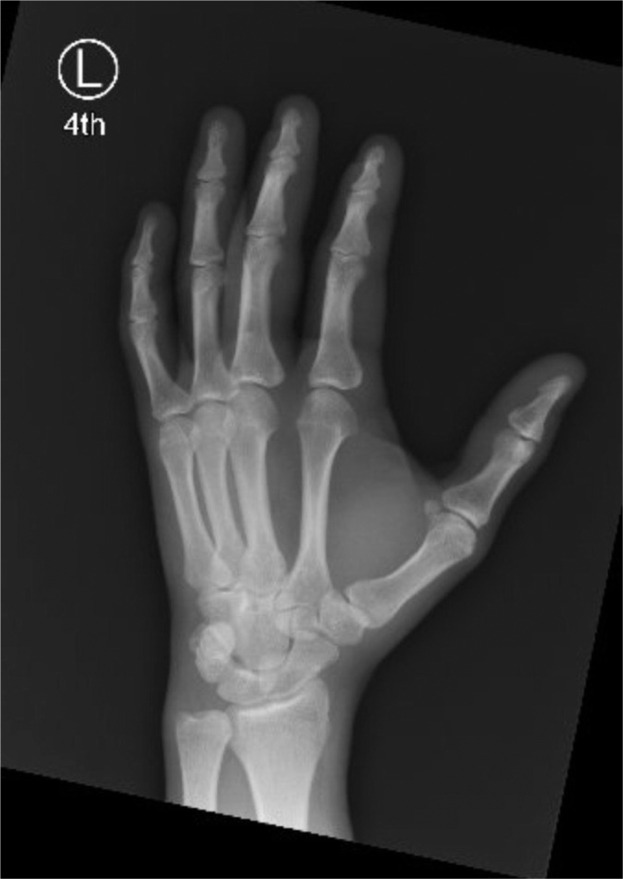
Oblique X-Ray of right hand (Case 2: Pre-op).
